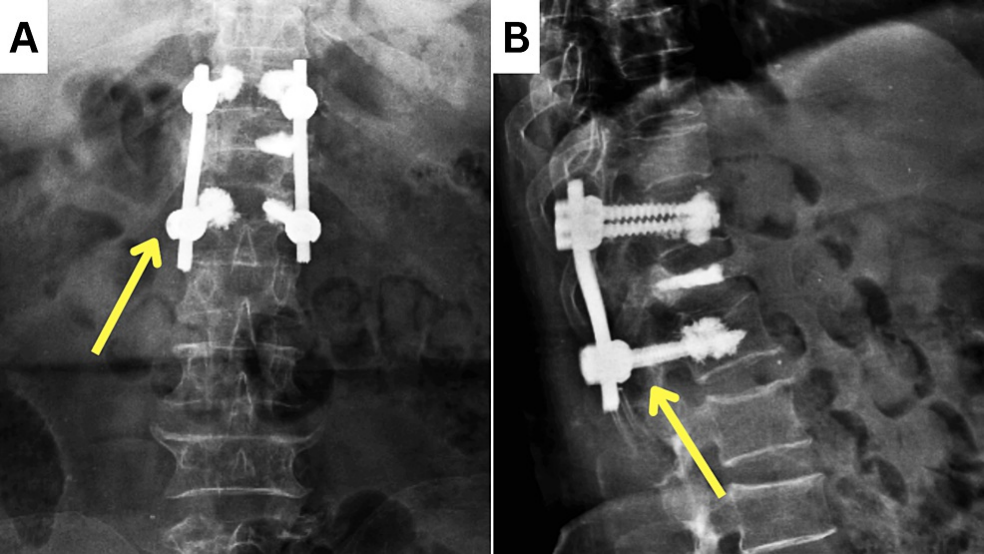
Post-operative X-ray of the spine The yellow arrow depicts the pedicle screw and connecting rods attaching D11 to the L1 vertebra. A: anterior view; B: lateral view
